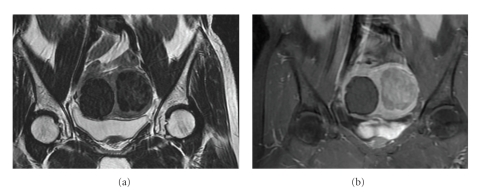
Screening MR images: (a) coronal T2-weighted image showing two fibroids, (b) coronal T1-weighted contrast-enhanced image—the right fibroid is already nonenhancing, whereas the left one is still viable.
